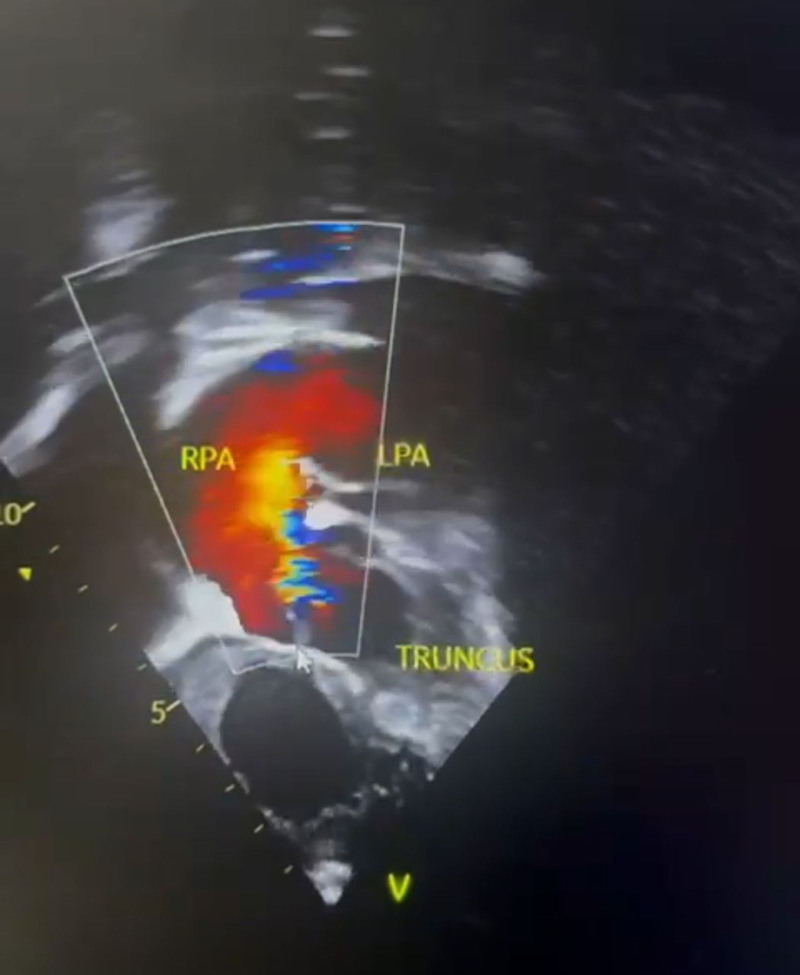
Doppler echocardiographic image showing severity of the ventricular septal defect. LPA = left pulmonary artery, RPA = right pulmonary artery.
